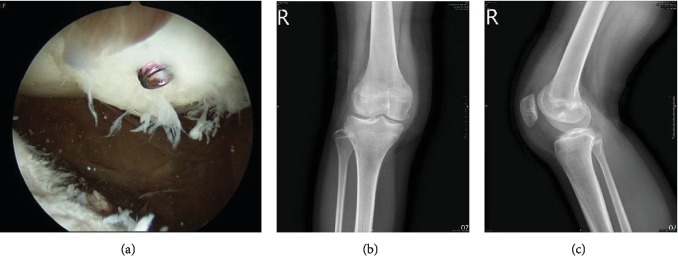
(a) Postoperative second-look arthroscopy at 1-year follow-up showing blurring of the cartilage and metallosis but no obvious degenerative changes in the cartilage. (b, c) Postoperative radiograph shows the healed fracture.
